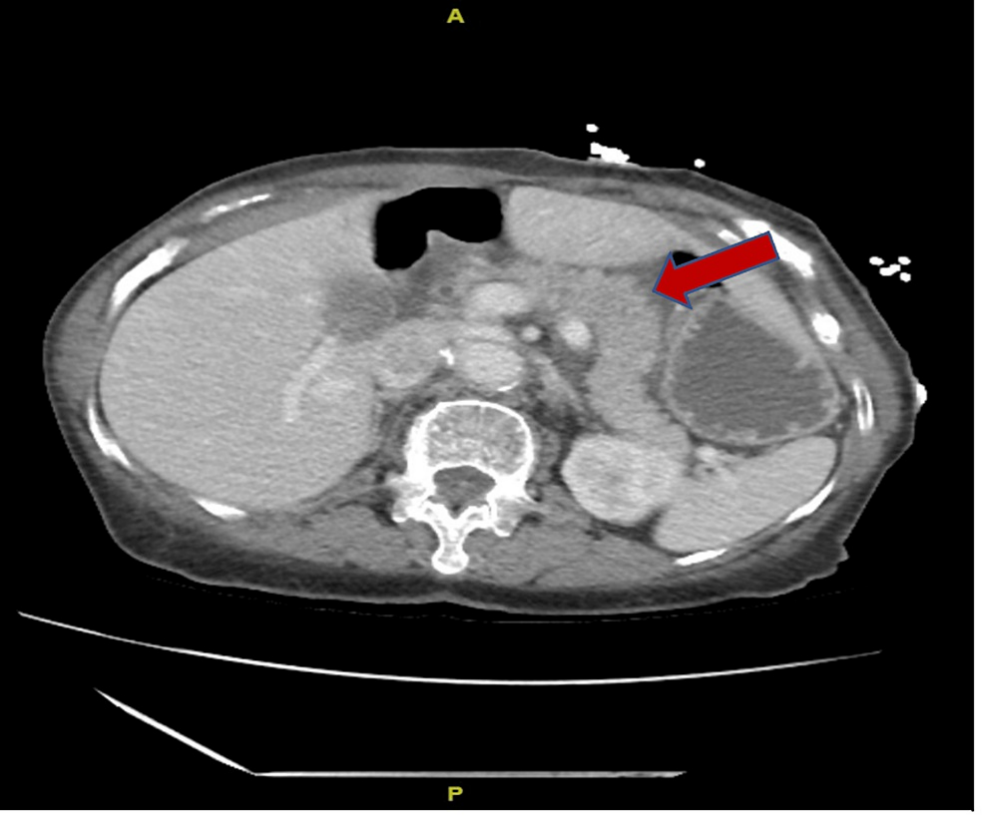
CT of the abdomen and pelvis without contrast revealing evidence of an edematous pancreas with peripancreatic fluid and fat stranding.
